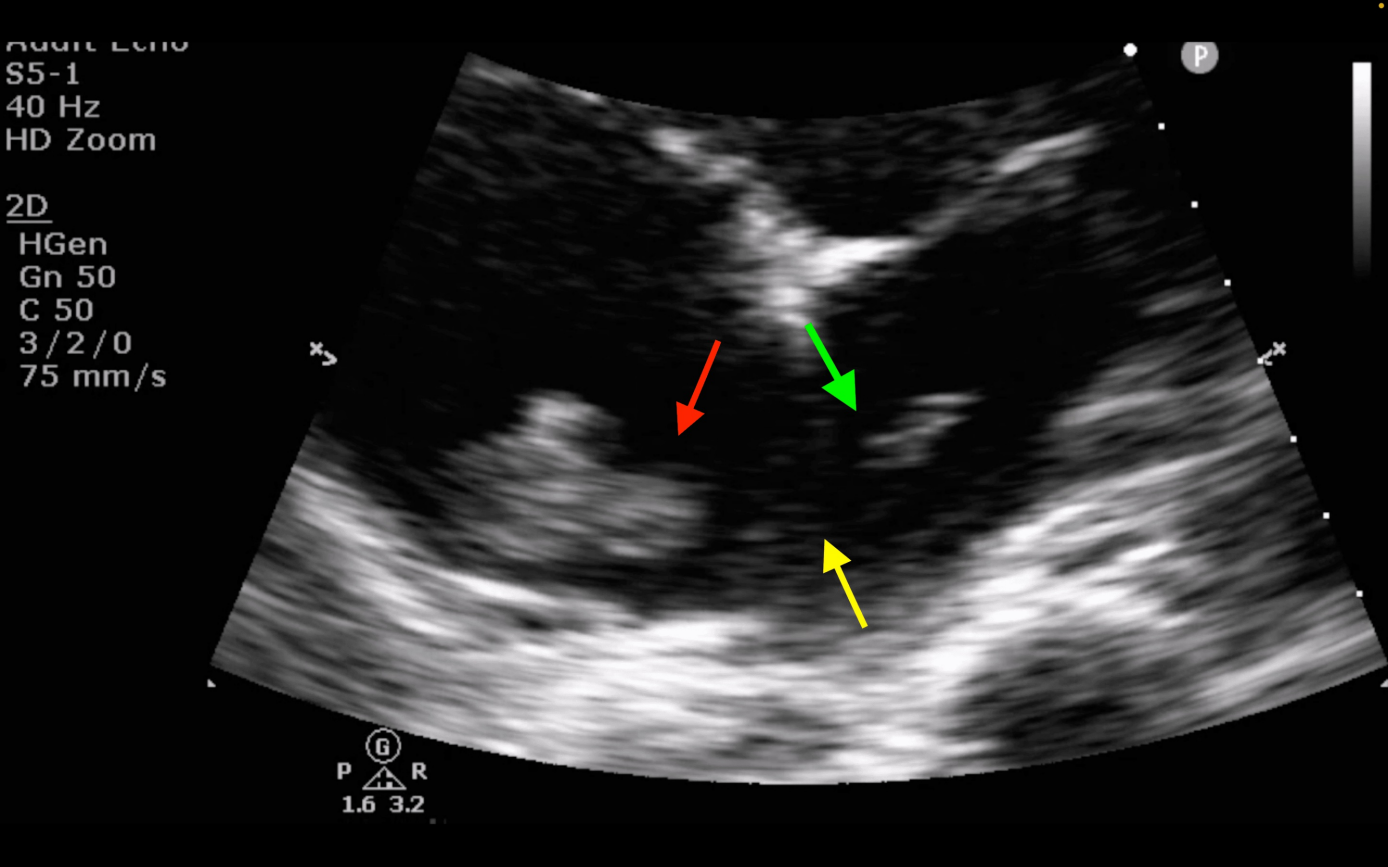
TTE showing a hyperechoic mass in the right atrium (red arrow) passing through the interatrial septum (yellow arrow) and appearing in the left atrium (green arrow), suggestive of a TSPFO TSPFO, thrombus straddling a patent foramen ovale; TTE, transthoracic echocardiogram
